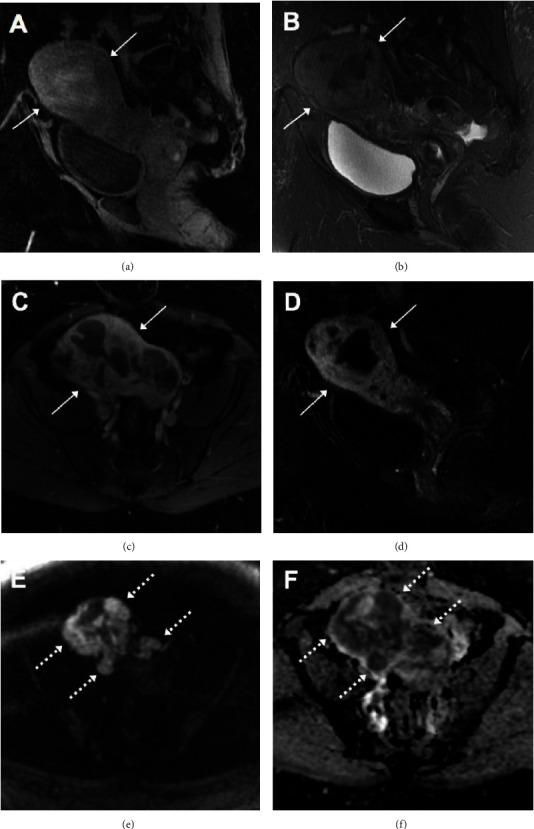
An example of pelvic MRI in a patient with high-grade uterine sarcoma (not the case presented in this study). The sagittal precontrast T1-weighted (a) and T2-weighted (b) MRI images demonstrate a heterogeneous mass (solid arrows, (a–d)) centered within the uterus which demonstrates enhancement on postcontrast axial (c) and sagittal (d) T1-weighted fat-saturated images. There are areas with restricted diffusion within the mass (dotted arrows, (e, f)) demonstrating high signal on DWI (e) with corresponding low signal on the ADC map (f).
